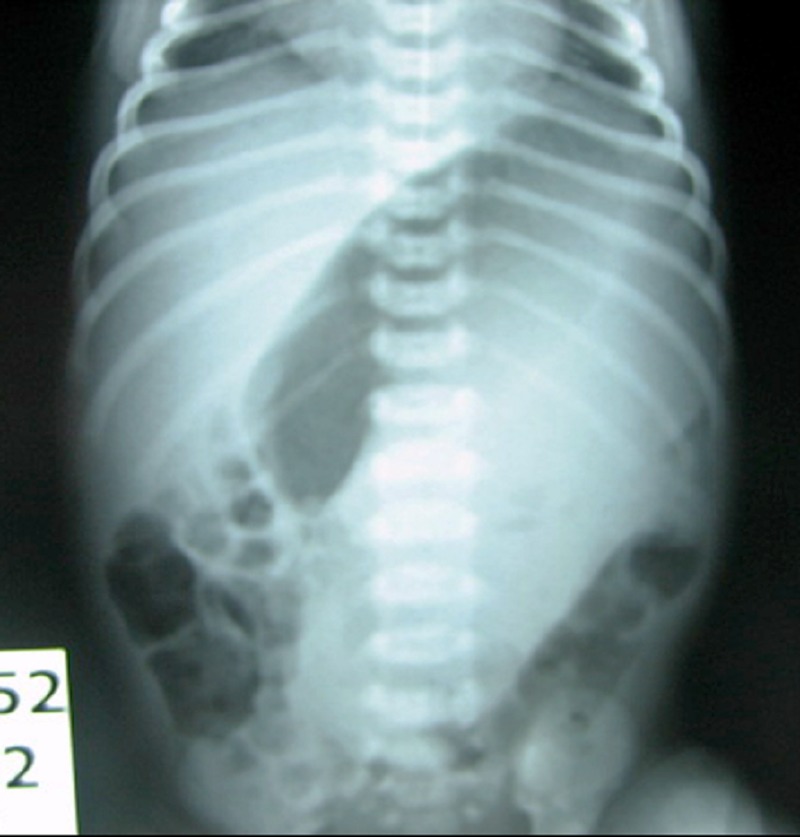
Figure 1: Plain film of abdomen showing gastric displacement by the mass.
